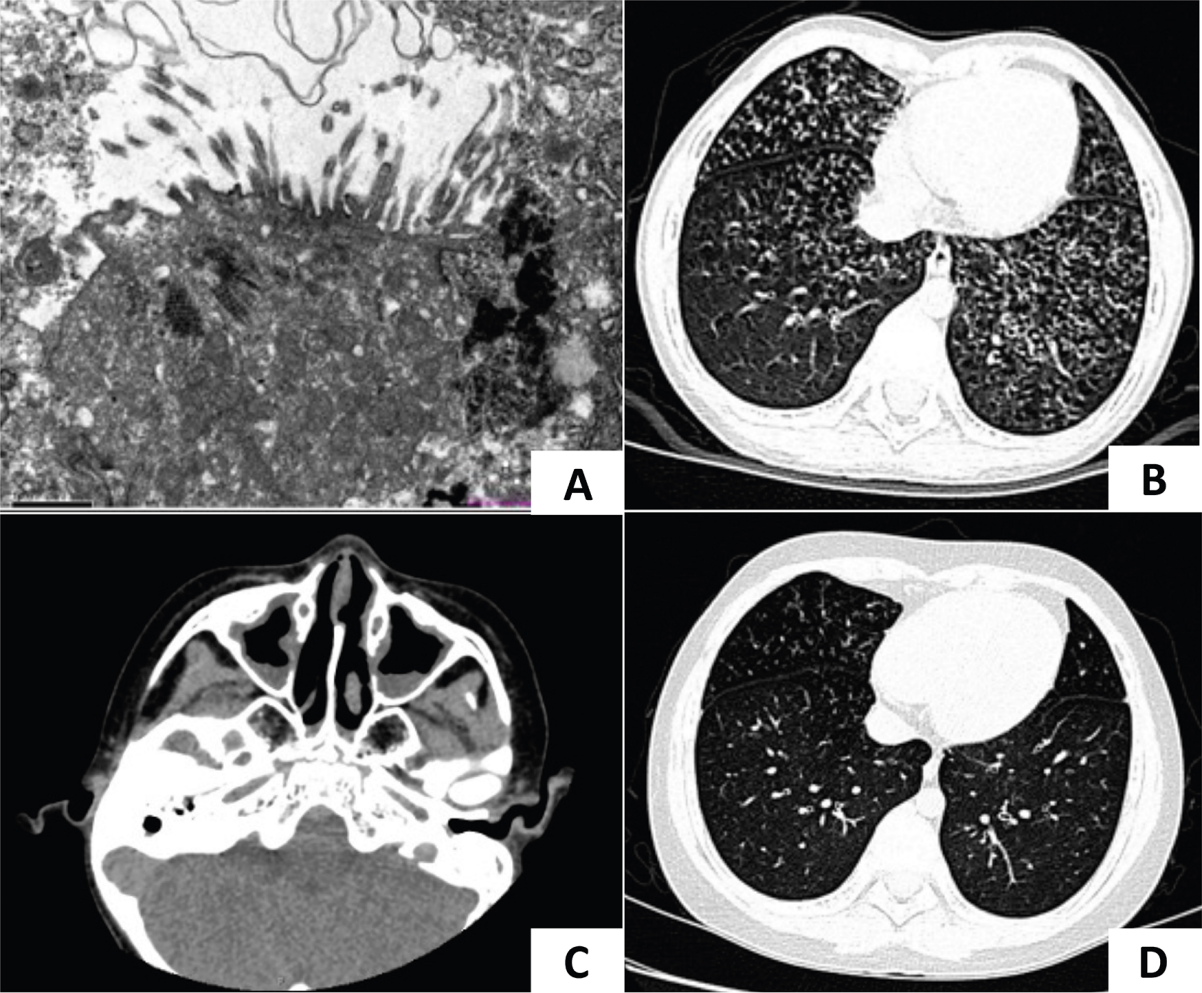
CT imaging and TEM of case 1. (A) TEM of case 1 showed microvilli on the epithelial surface, inflammatory cell infiltration, and absent cilia in multiple sections. (B) A lung CT scan identified bilateral diffuse micronodules and “tree-in-bud” signs. (C) A sinus CT scan showed mucosal thickening of bilateral nasal cavities and maxillary sinuses. (D) Lesions in the lower lungs improved after treatment.
